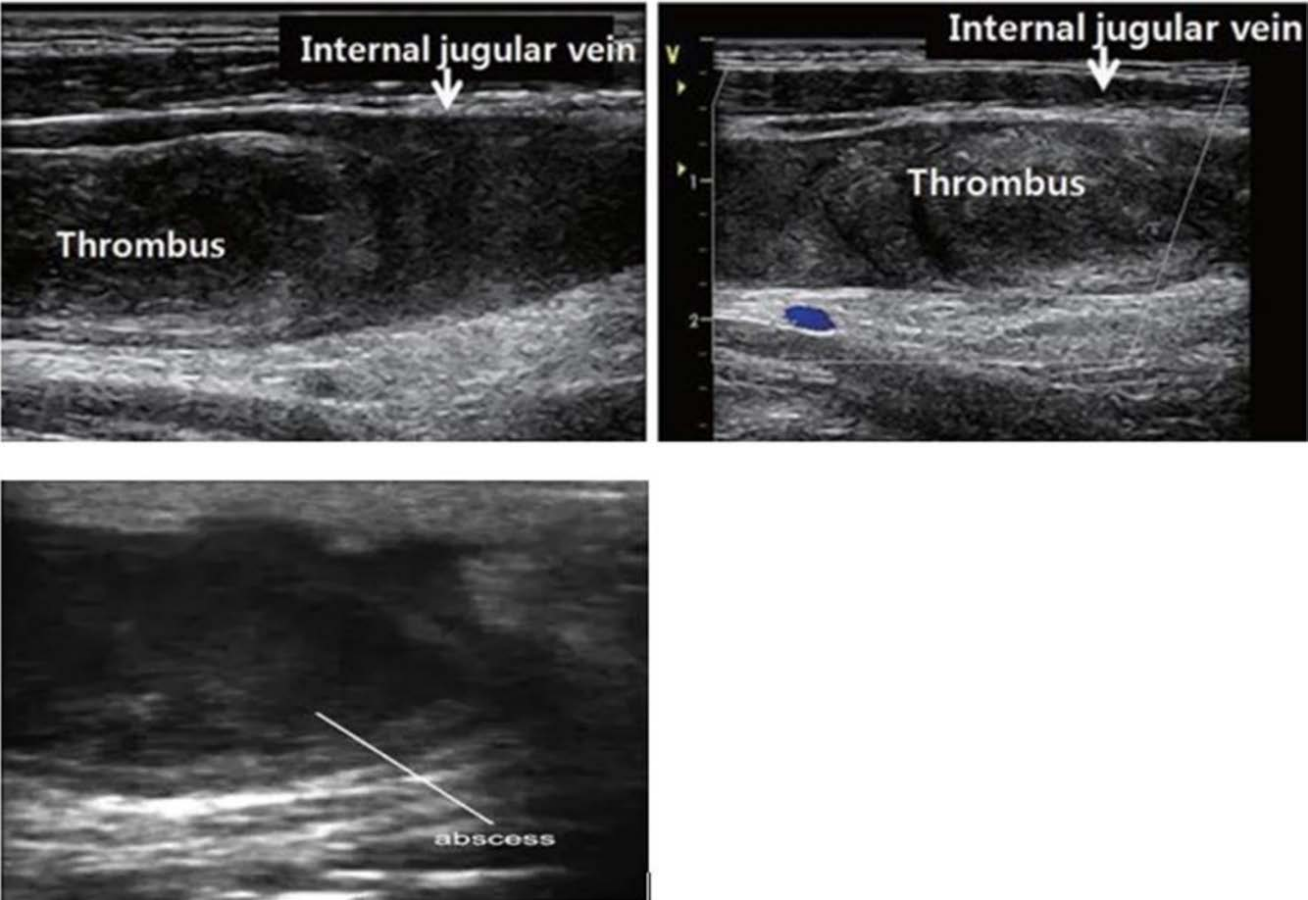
The longitudinal image of the left neck ultrasound shows thrombosis of the left IJV with partial obstruction of the lumen, color flow mapping shows no flow. In addition, 3.9 cm by 2.6 cm left anterolateral neck abscess posterolateral to sternocleidomastoid muscle is seen. IJV = internal jugular vein.
